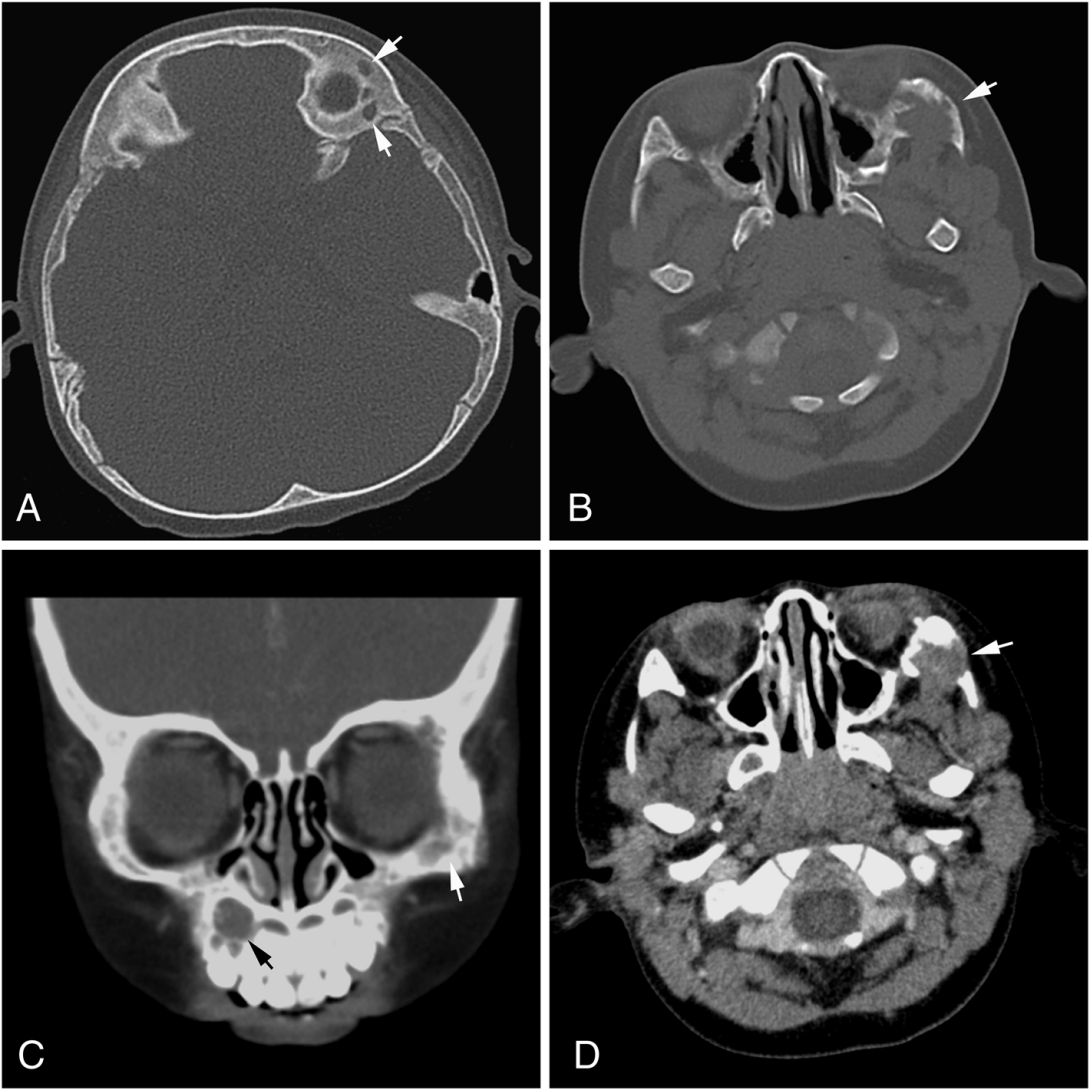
Radiographic examination of the lesions. (A) Cmputed tomography (CT) scan showed osteolytic lesions of the left orbital bone (white arrow). (B) A osteolytic lesion of the left zygomatic bone (white arrow) appeared to have an indistinct border in periphery. (C) Coronal CT scan showed multiple osseous destruction of maxillary bone (black arrow) and left zygomatic bone (white arrow). (D) Postcontrast axial CT scan in soft tissue windows revealed that an irregular mass destroyed the left zygomatic bone (white arrow).
